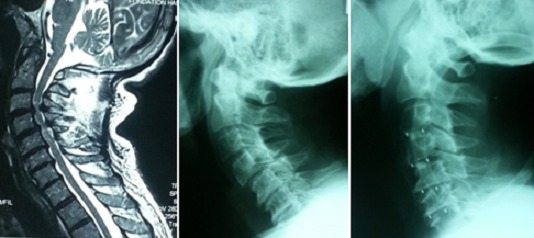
IRM préopératoire coupe sagittale en séquence pondérée T2 objectivant une myélopathie cervicarthrosique avec hyper signal médullaire en regard de C5, radiographie préopératoire et à 12 mois postopératoire d'un patient de 52 ans ayant bénéficié d'une dissectomie de 4 niveaux avec mise en place de 4 cages
